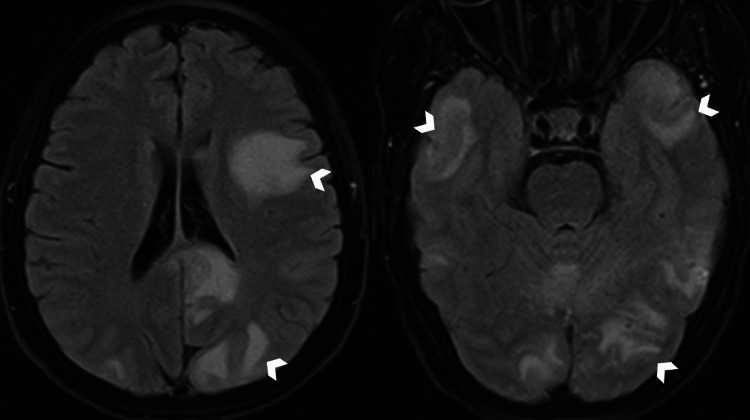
Axial slices in the FLAIR sequence showing hyperintensities in bilateral subcortical and periventricular hemispheric white matter, involving U-fibers and the splenium of the corpus callosum. FLAIR: fluid-attenuated inversion recovery.
